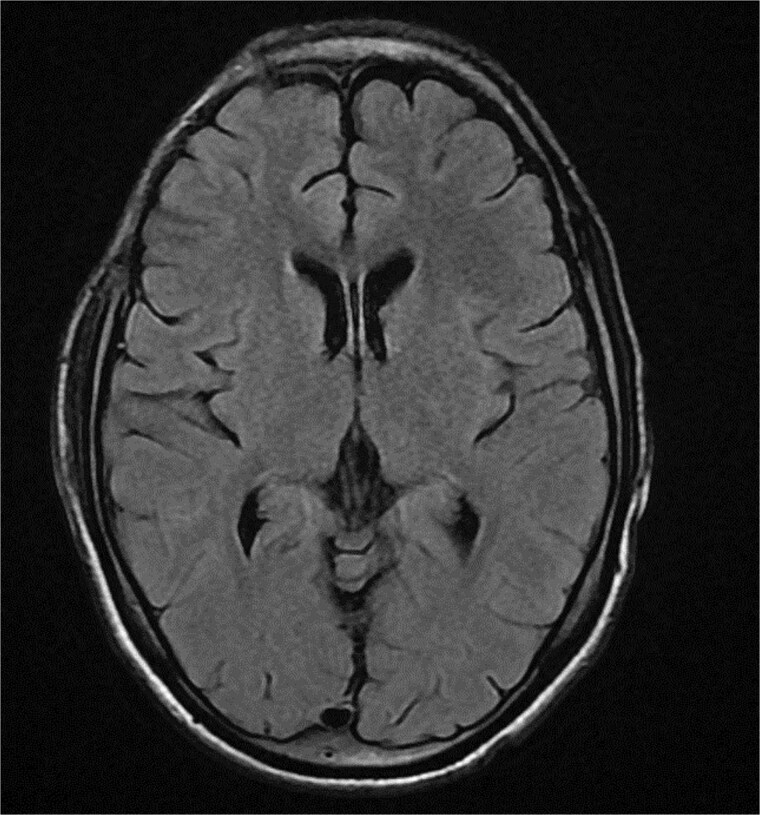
Axial FLAIR MRI at 1 month postoperatively showing the right frontal craniectomy flap with complete resolution of the cerebral empyema.
